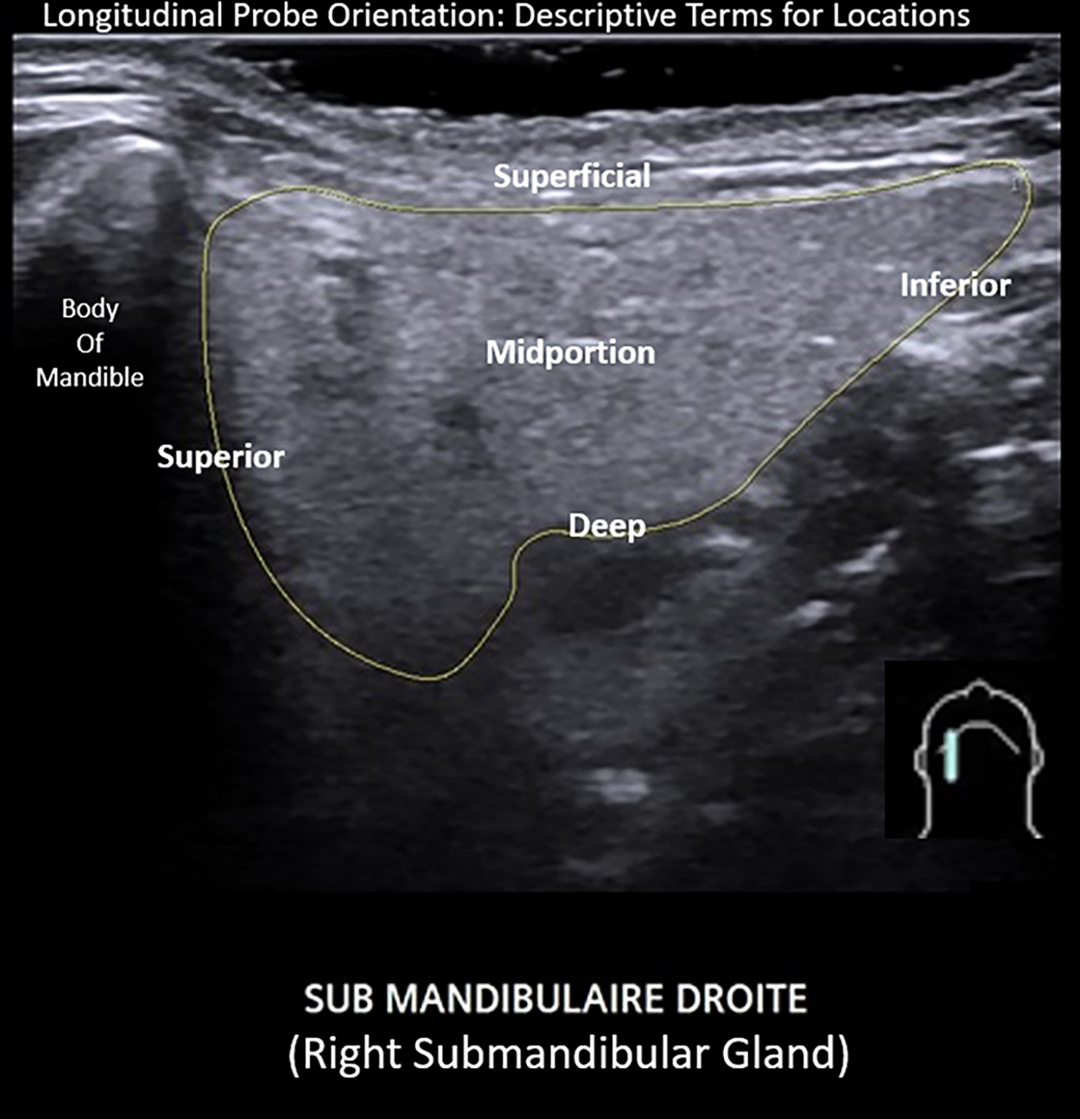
Ultrasound image employing longitudinal orientation of the probe identifies regions within the outlined right submandibular gland that are labeled with descriptive terms (image courtesy of Dr. Philippe Katz; relabeled).
